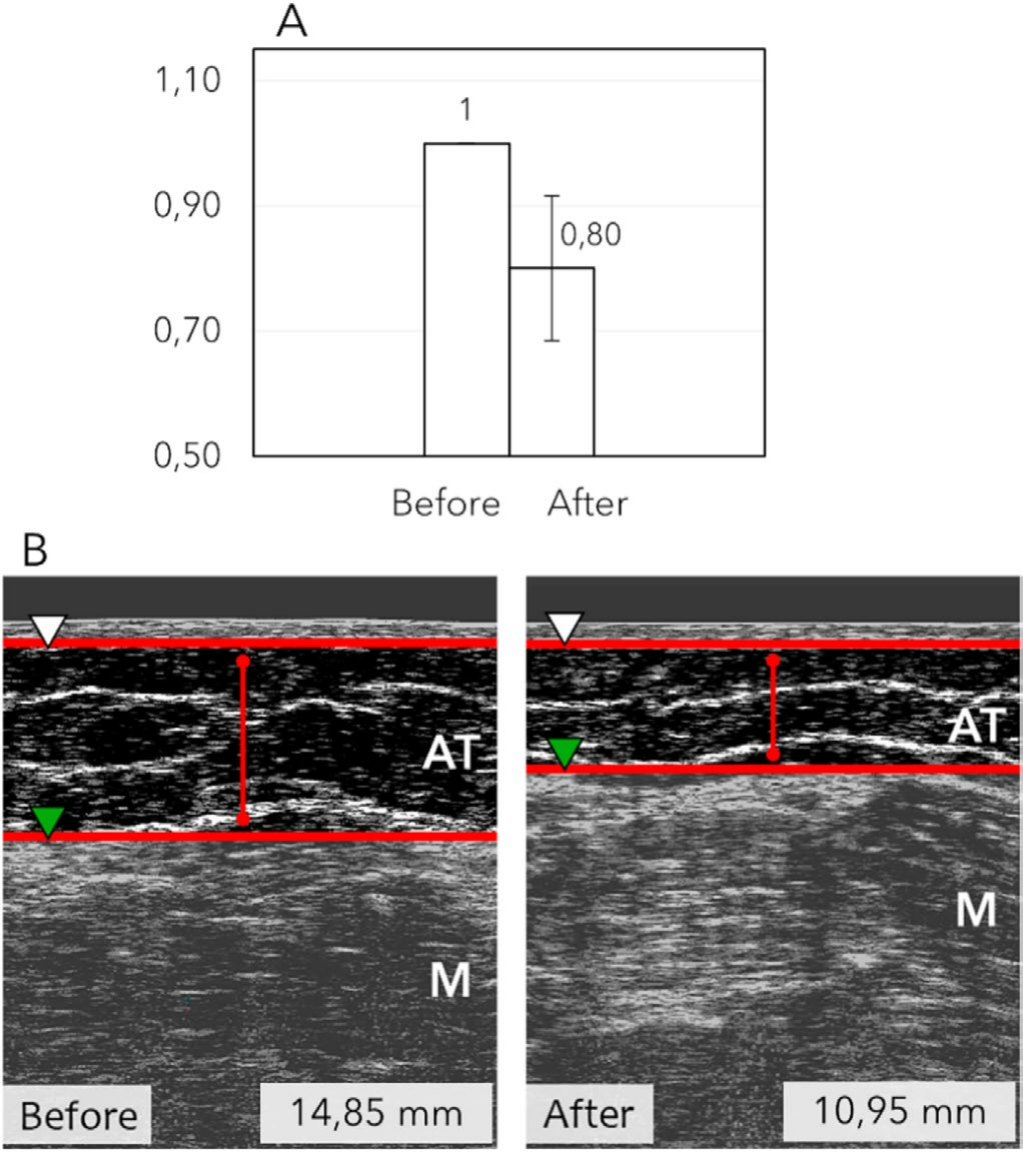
Ultrasound evaluation of subcutaneous fat layer thickness in the thighs after 8 Symmed sessions (*n* = 13). (A) Normalized representations of the average fat layer thickness reduction. (B) Example of subcutaneous ultrasound images showing a fat thickness decrease of 3.90 mm. ▷: deep dermis line;
